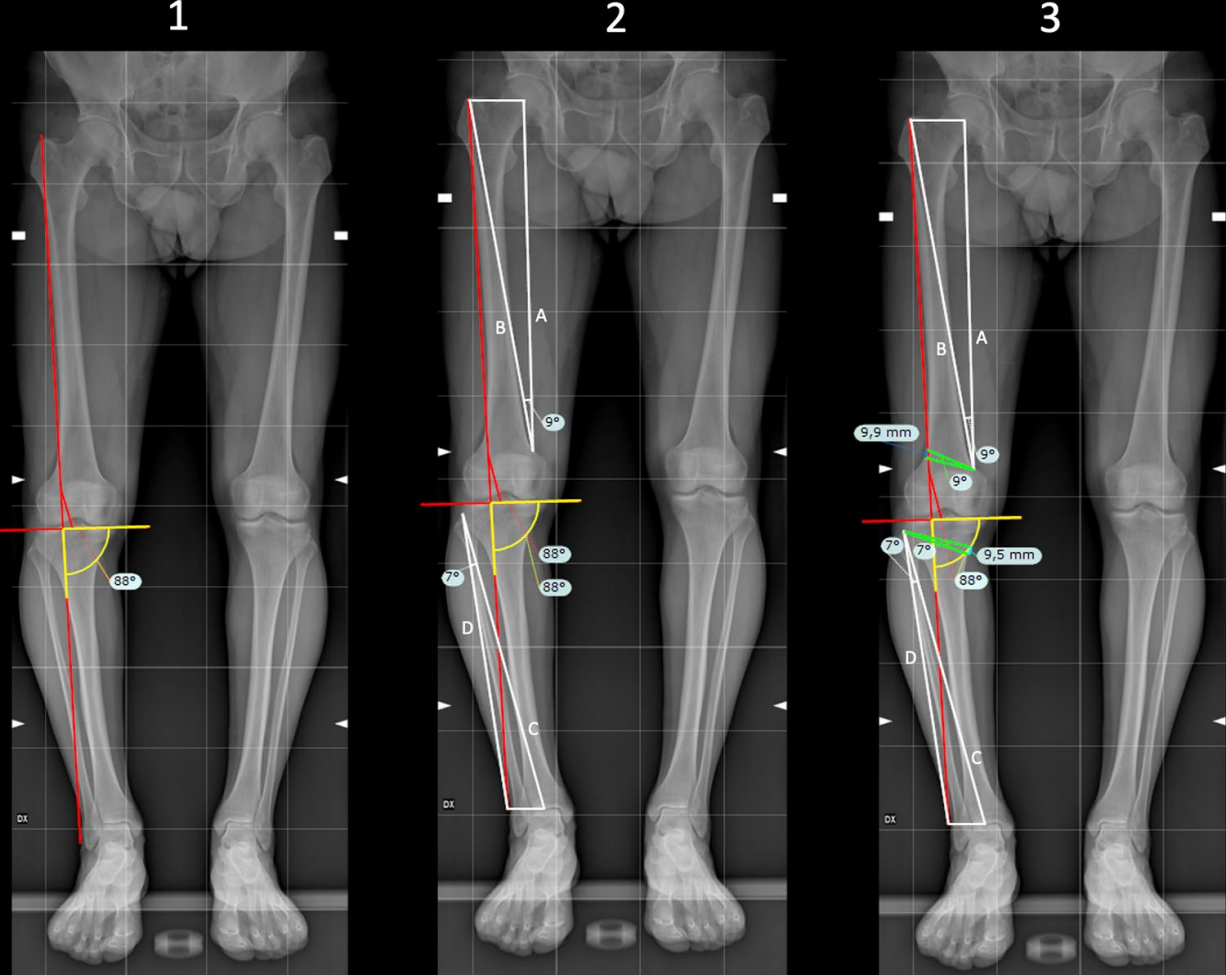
Bilateral weight-bearing full-length AP digital radiograph for DLO planning according to the authors’ proposed method. 1.1 A 88 degrees of NM-JL value is established. Determine a correction point at the knee joint line at 62.5% of the tibial plateau width measured from the medial tibial cortex. Draw the new loading axis passing through the correction point defined. 1.2 The hinge point for the osteotomies was determined. At the femoral level, an A-line connects the femoral hinge point and the hip centre. At the same time, a B-line connects the femoral hinge point and the intersection point of the new loading axis at the femoral level. A C-line connects the tibial hinge point and the centre of the ankle joint at the tibial level. Finally, a D-line connects the tibial hinge point and the intersection point of the new loading axis at the tibial level. 1.3 The angle between these lines represents the femoral correction angle (the green angle at femoral level) and the tibial correction angle (the green angle at tibia level.) The osteotomies’ millimetre open and closure gaps were measured (blue lines at femoral and tibial levels)
